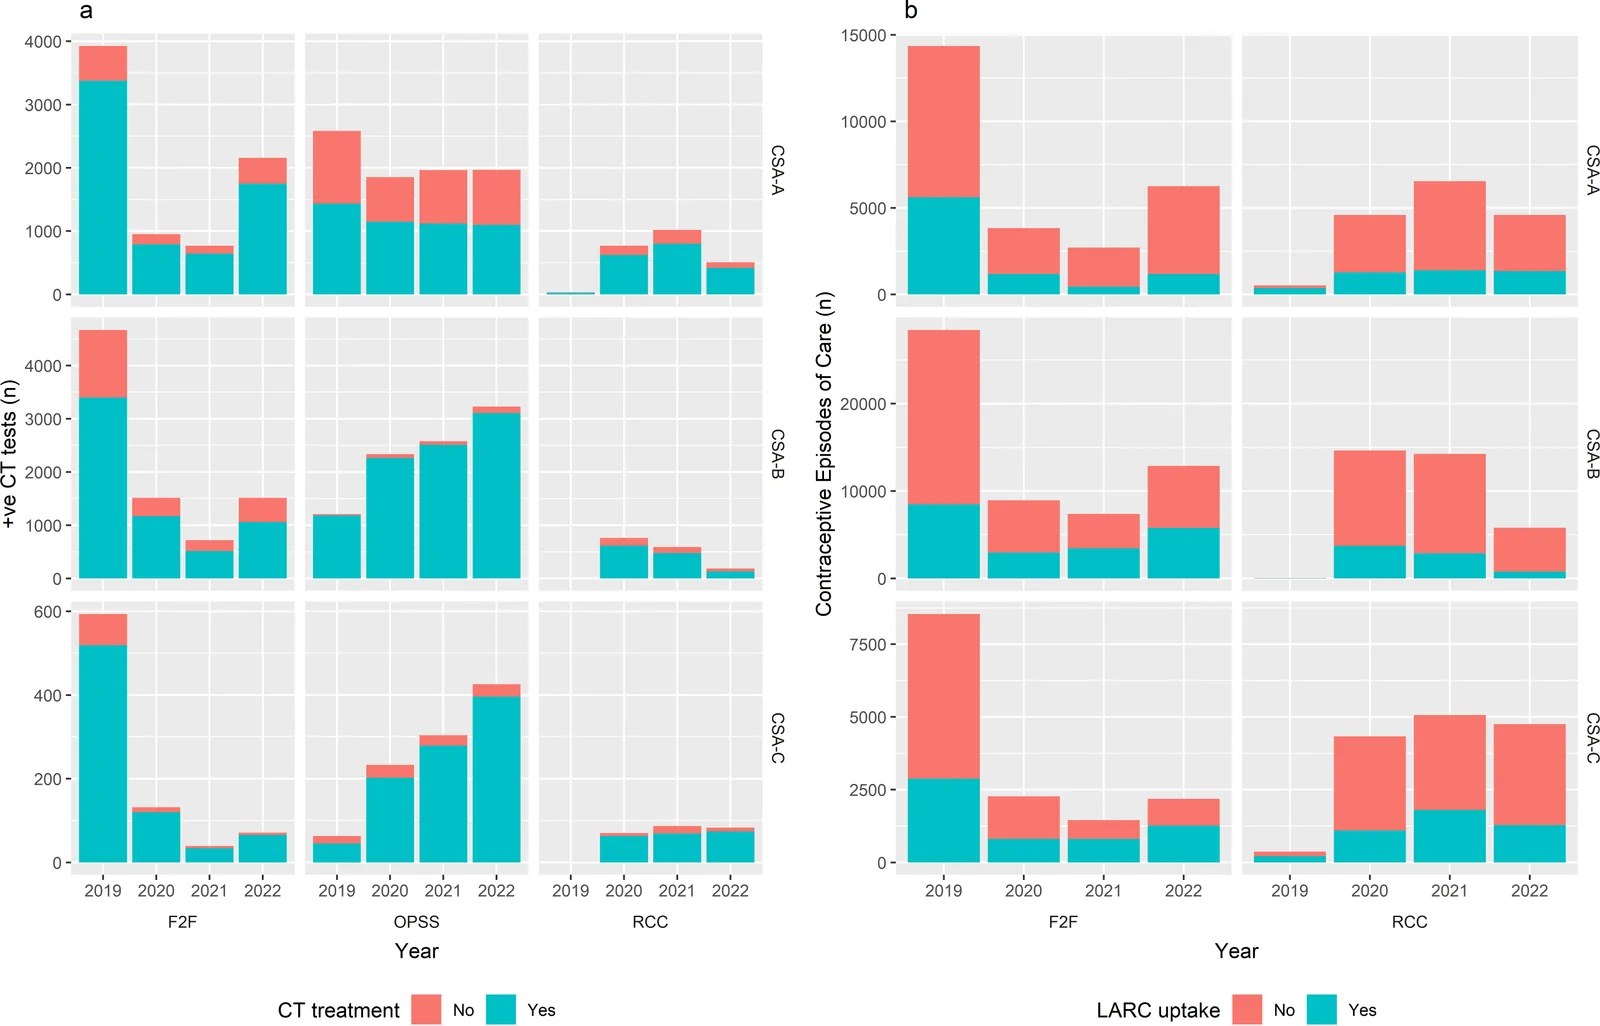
(a) Diagnosed cases of chlamydia (CT) and summary of treatment success (within 6 weeks) in 2019–2022. Rows correspond to the three case study areas (CSA-A-C), and data for each outcome are also split according to whether the initial contact in each 6-week episode of care was: face-to-face (F2F), online postal self-sampling (OPSS) or a remote telephone or video clinical consultation (RCC). (b) Total counts of episodes of care involving any contraceptive care among women aged 16–49 years in 2019–2022, and proportion of these episodes in which there was any record of new or adjusted long-acting contraception (LARC). Rows correspond to the three CSA-A-C, and data for each outcome are also split according to whether the initial contact in each 12-week episode of care was F2F or RCC.
